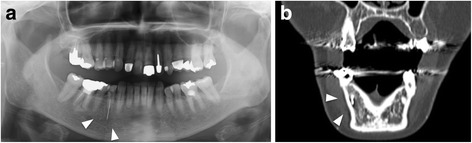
a Panoramic radiographs revealed a well-defined fractured instrument lying in the mandible beyond the apical foramen of the right mandibular premolar tooth. b The computed tomography image revealed that the fractured segment was located in the mandibular bone
